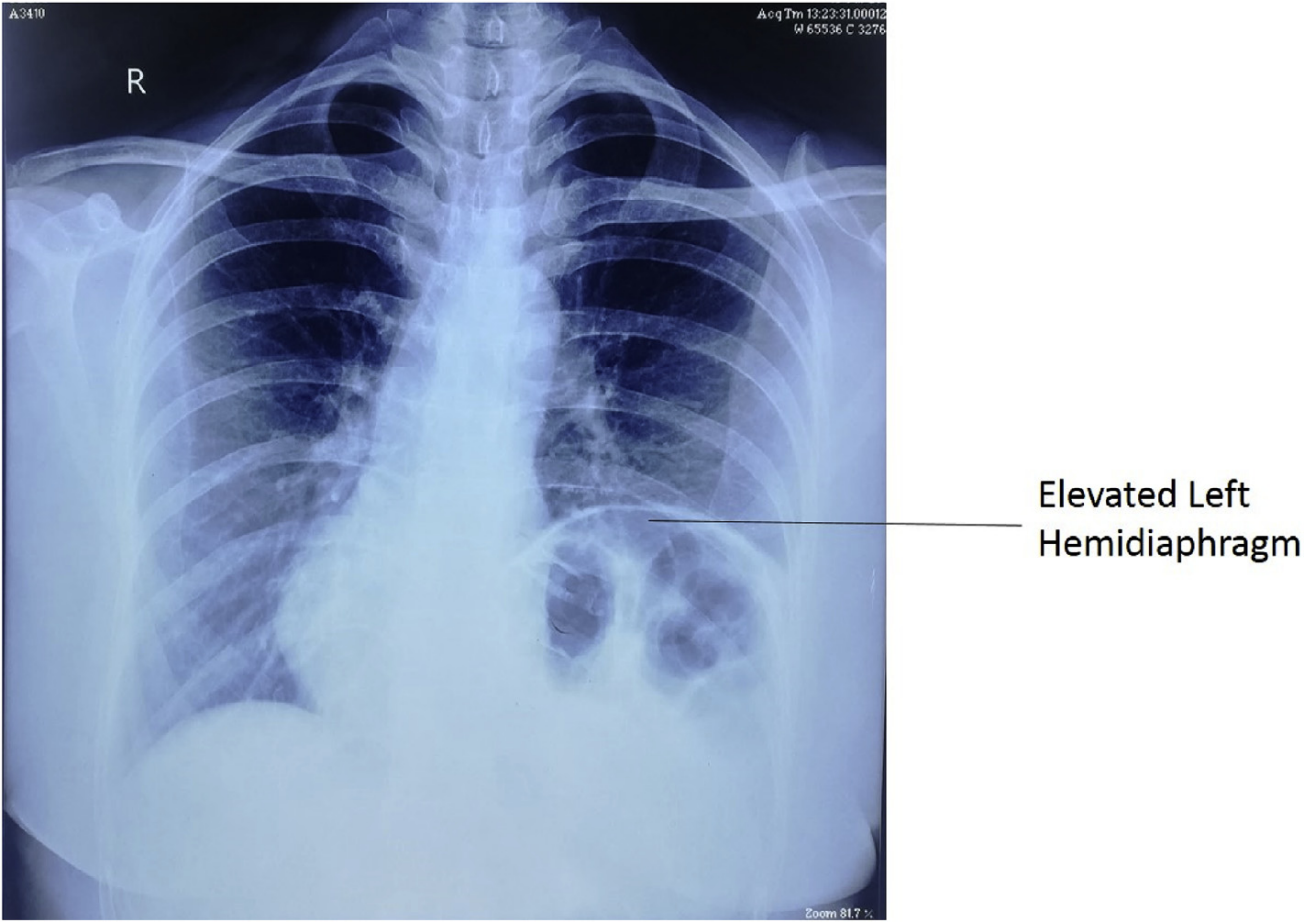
Chest X-ray showing elevated left hemidiaphragm up to 4th rib with normal gas shadow of bowel underneath and mediastinal shift towards the right side.
